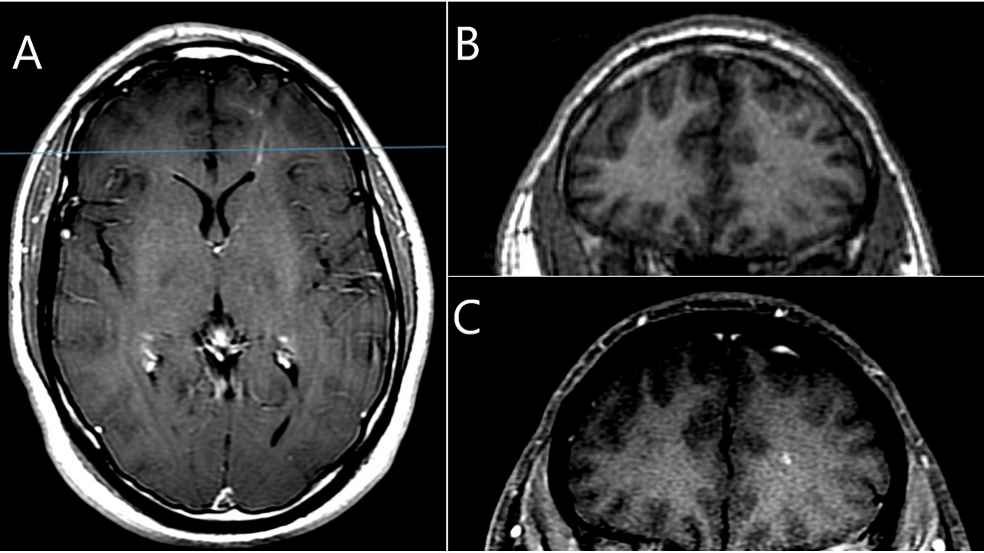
Brain magnetic resonance imaging with and without contrast identified few foci of punctate susceptibility abnormalities in the left frontal lobe (A) T1 axial sequence, contrast positive, blue line indicates area of coronal cross-section; (B) coronal SPGR sequence, contrast negative; (C) T1 coronal sequence, contrast positive.
